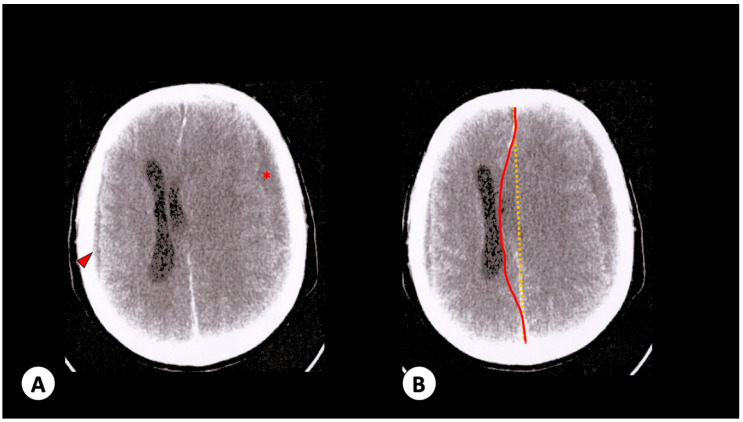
Patient 2 radiologic observations. (A) Brain no-contrast computed tomography with hypodense images in right parieto-occipital (0.5 cm—arrowhead) and left front-temporal (1.6 cm—red star) areas. (B) Corresponding brain contrasted computed tomography with middle-line deviation (red line) compared to regular condition (yellow line). It is not possible to view left lateral ventricular and cerebellar cisterns due to compression by left subarachnoid hemorrhage.
